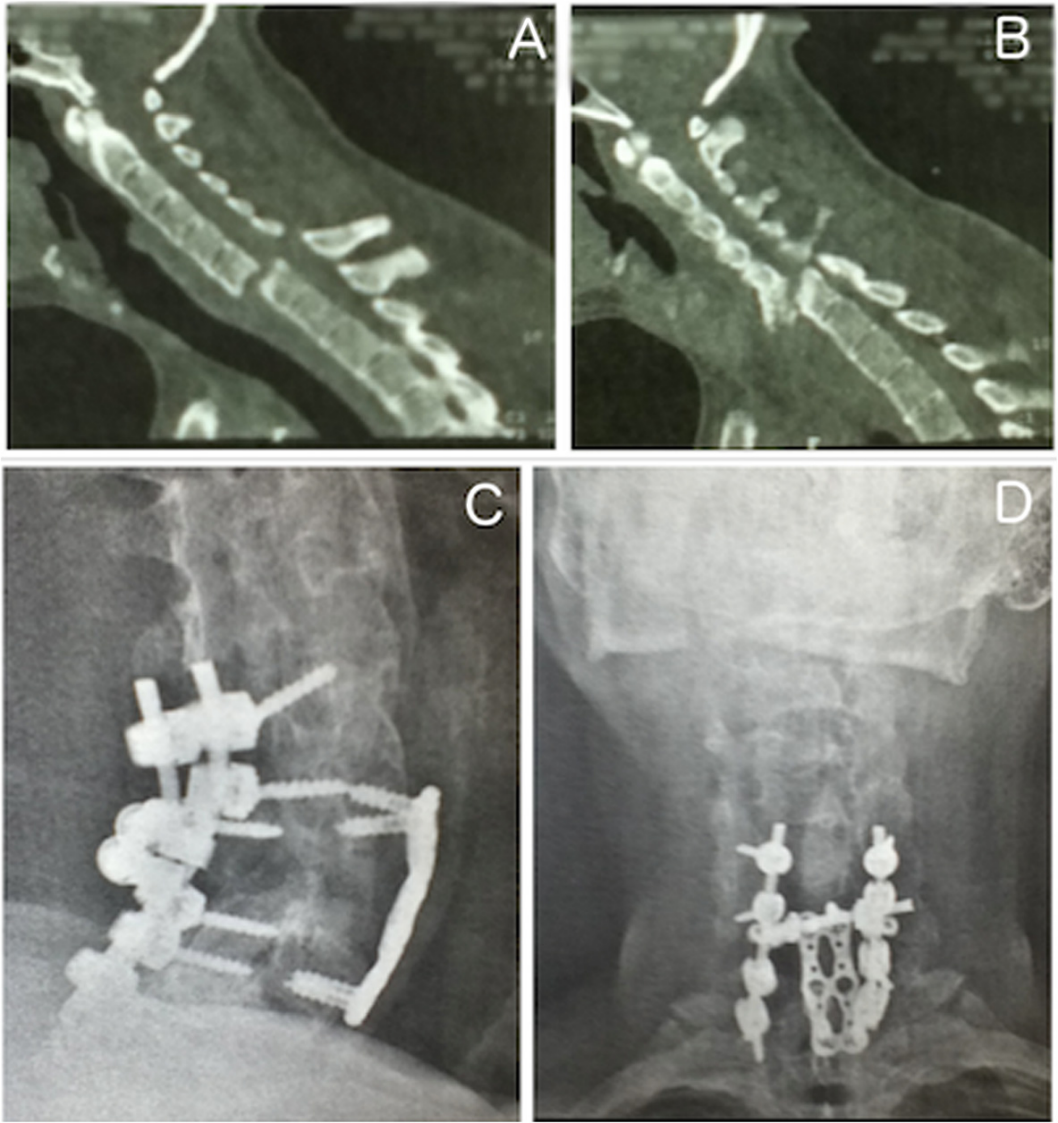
Clinical and surgical presentation of Patient 3. Observe in (a) and (b) the sagittal computed tomography scan images showing ankylosed cervical spine and a fracture-dislocation of C6 to C7. In (c) and (d), the radiographic images in sagittal (c) and posteroanterior (d) views demonstrating the stabilization in 360 degrees
